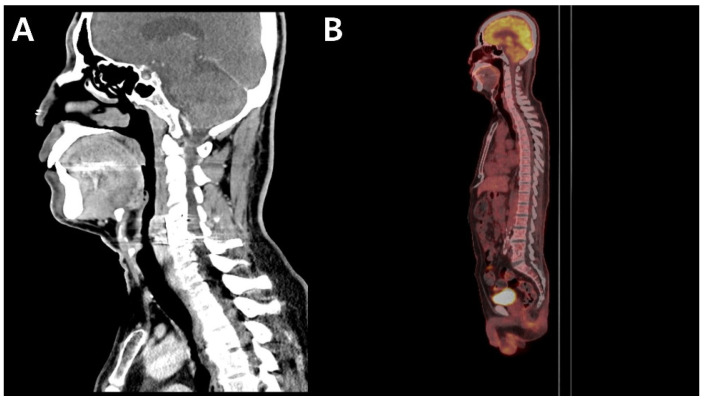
Follow-up imaging demonstrating the patient’s clinical outcome following the bedaquiline-containing anti-tuberculosis therapy: (A) Follow-up neck CT demonstrating resolution of the prevertebral and epidural abscesses. (B) Follow-up PET-CT demonstrating complete disappearance of FDG uptake in the vertebral bodies and prevertebral space.
